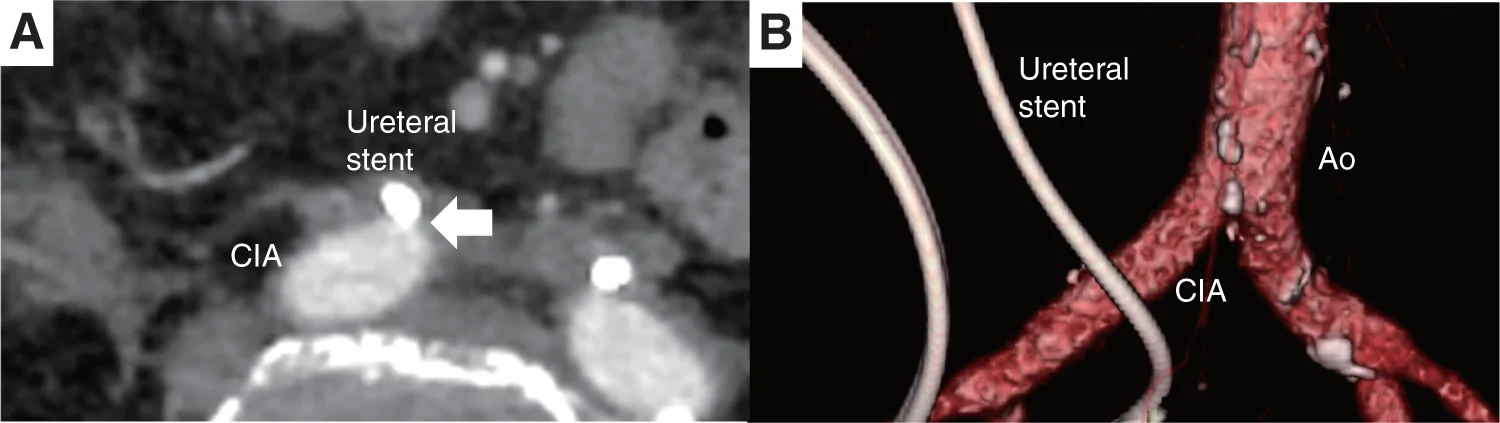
Contrast-enhanced CT findings suggestive of uretero-arterial fistula. (A) Axial CT image demonstrating close proximity between the right ureteral stent and the right CIA at the crossing point. (B) Three-dimensional reconstructed CT image showing the spatial relationship between the right ureteral stent and the right CIA. Ao, aorta; CIA, common iliac artery; CT, computed tomography
